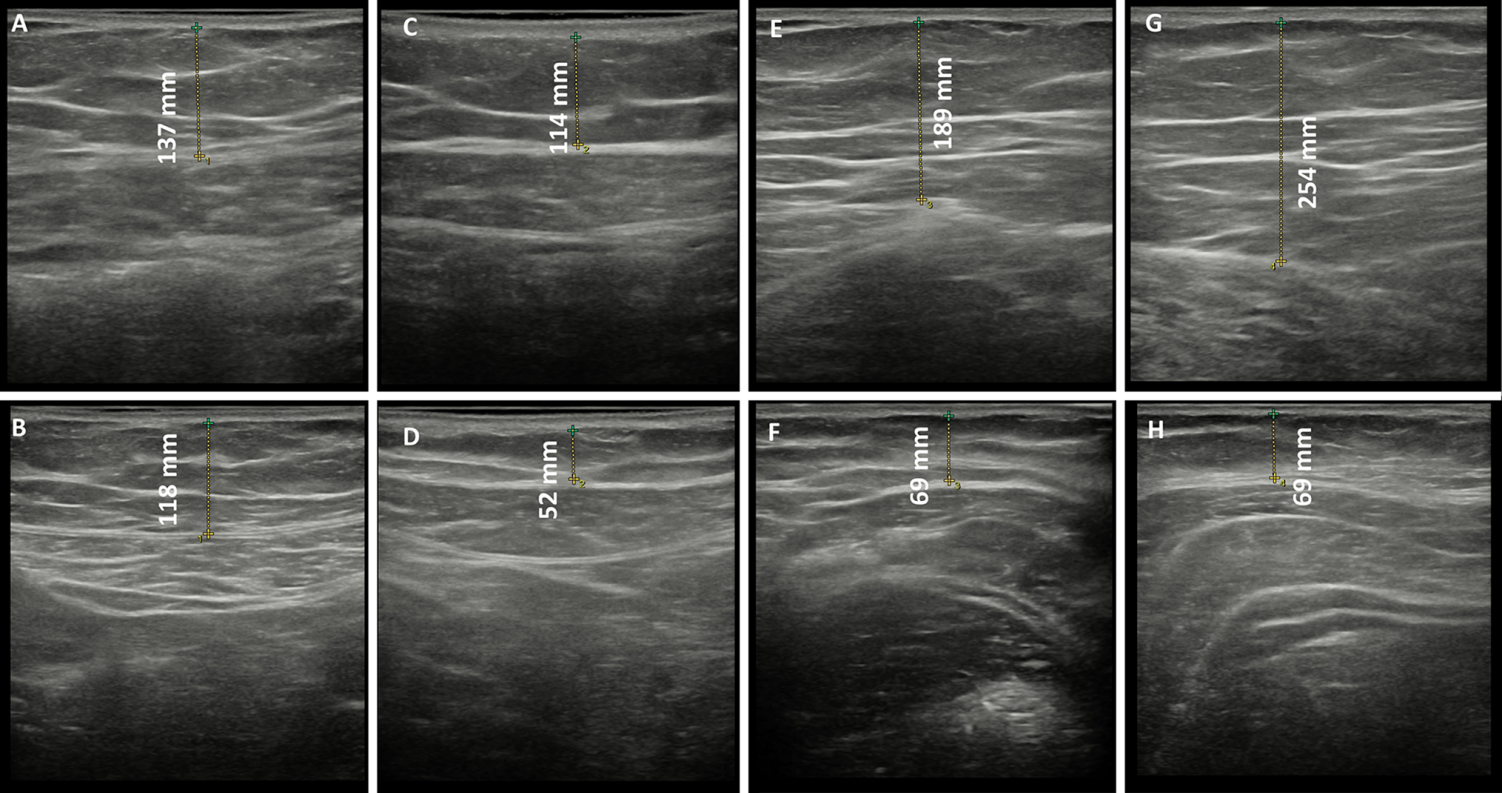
Ultrasound imaging of a 34-year-old woman who underwent four sessions in abdomen and hips with the new non-invasive body contouring device. A Infraumbilical thickness before treatment. B Infraumbilical thickness after treatment. C Supraumbilical thickness before treatment. D Supraumbilical thickness after treatment. E Right hip thickness before treatment. F Right hip thickness after treatment. G Left hip thickness before treatment. H Left hip thickness after treatment
